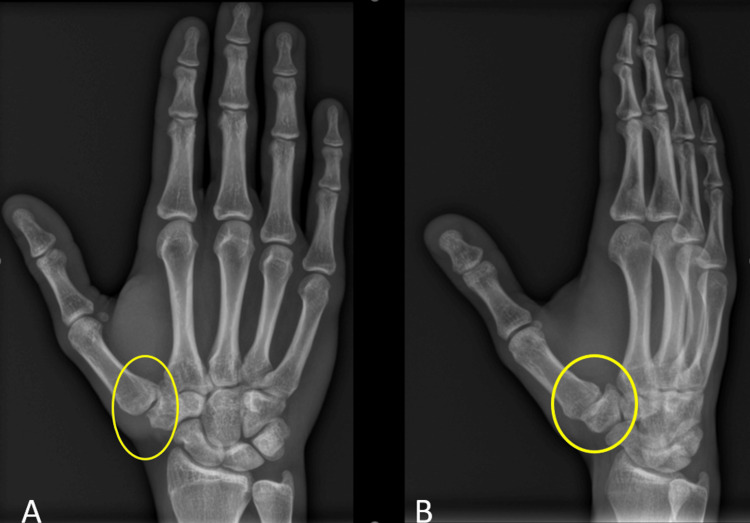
(A, B) Anteroposterior and oblique radiographs of the right hand three months postoperatively.
